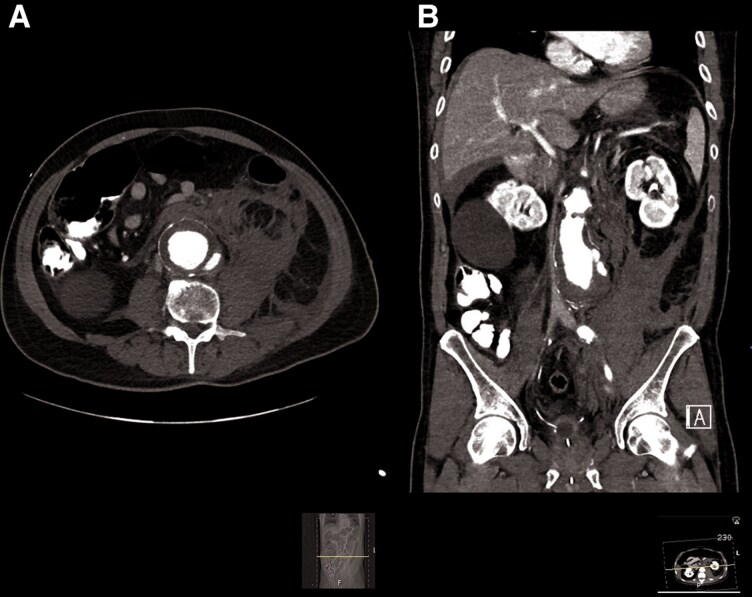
Abdominal CT showing a large infrarenal aortic aneurysm with mural thrombus and contrast extravasation through the posterior wall with extensive retroperitoneal haemorrhage.
